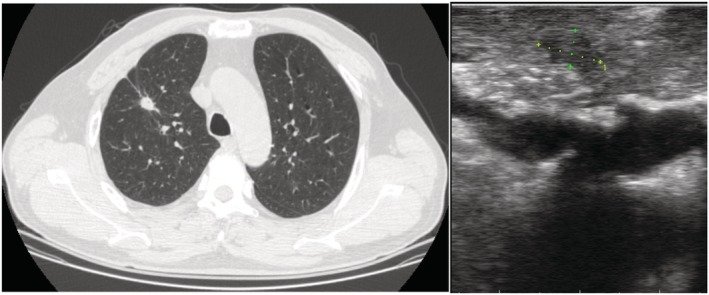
(a) CT scan and (b) ultrasound manifestations of pulmonary nodules.
